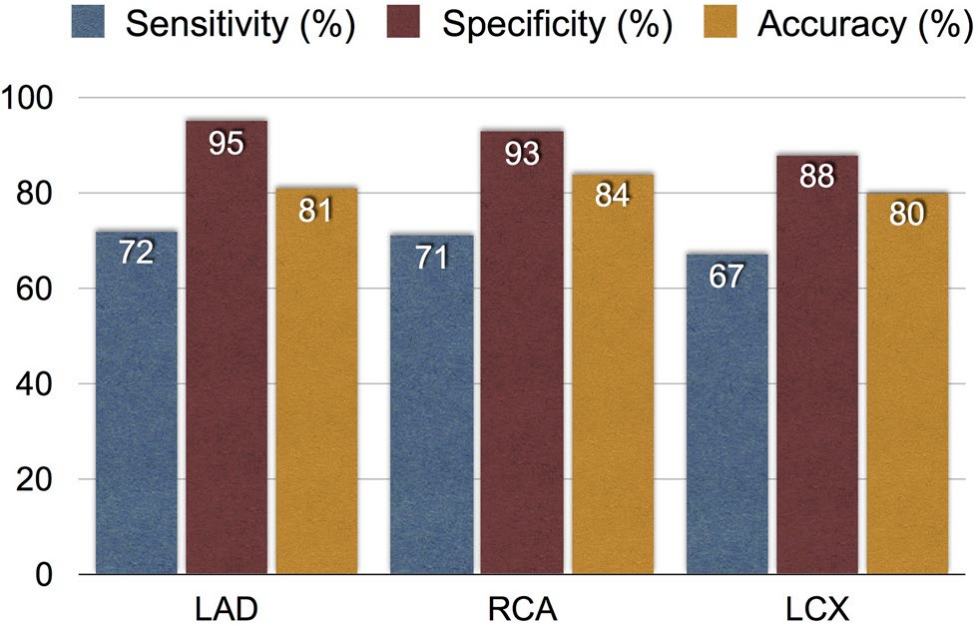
Diagnostic performance of stress myocardial perfusion imaging by IQ·SPECT using a prone position. Sensitivity, specificity and accuracy are shown in each coronary territory based on coronary angiography. *LAD* left anterior descending, *RCA* right coronary artery, *LCX* left circumflex
